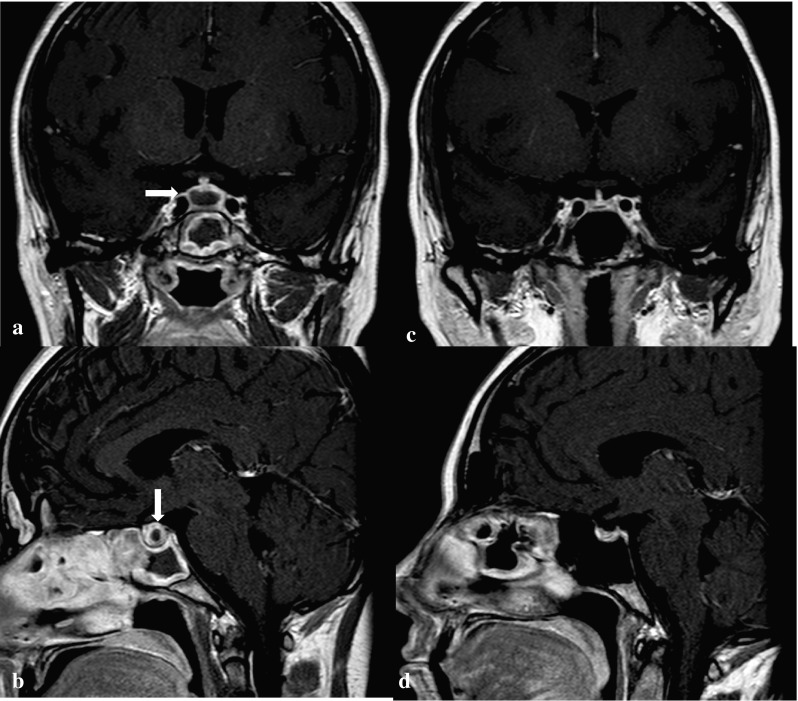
T1-weighted MRI (coronal and sagittal view) of a 39-year-old woman (Case 2) showing a sellar mass with central cystic lesion before (a and b) and after (c and d) conventional treatment for GPA associated PD
